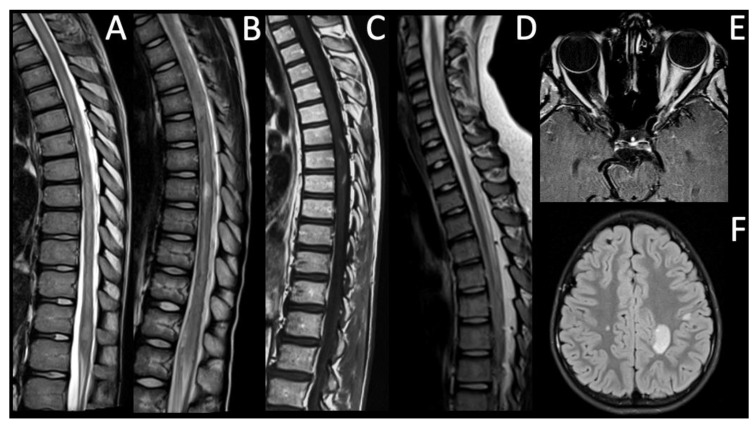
NMOSDs without AQP4-IgG. (A). Sagittal T2-WI shows multiple and confluent hyperintense lesions in the cervical and dorsal spine consistent with LETM (case 4), (B). sagittal T2-WI shows multiple and confluent hyperintense lesions in the dorsal spine extending from level T5 to the conus medullaris (case 5), (C). sagittal T1-WI acquired after the intravenous (iv) administration of gadolinium (gd) shows intramedullary enhancement between T6 and T8 levels (case 6), (D). sagittal T2-WI shows hyperintense lesions in the cervical spine (C2–C7) consistent with LTM (case 7), (E). axial T1-WI acquired after iv administration of gd shows left optic nerve enhancement consistent with left ON (case 7), (F) axial T2-FLAIR shows multiple brain hyperintense lesions (case 5).
